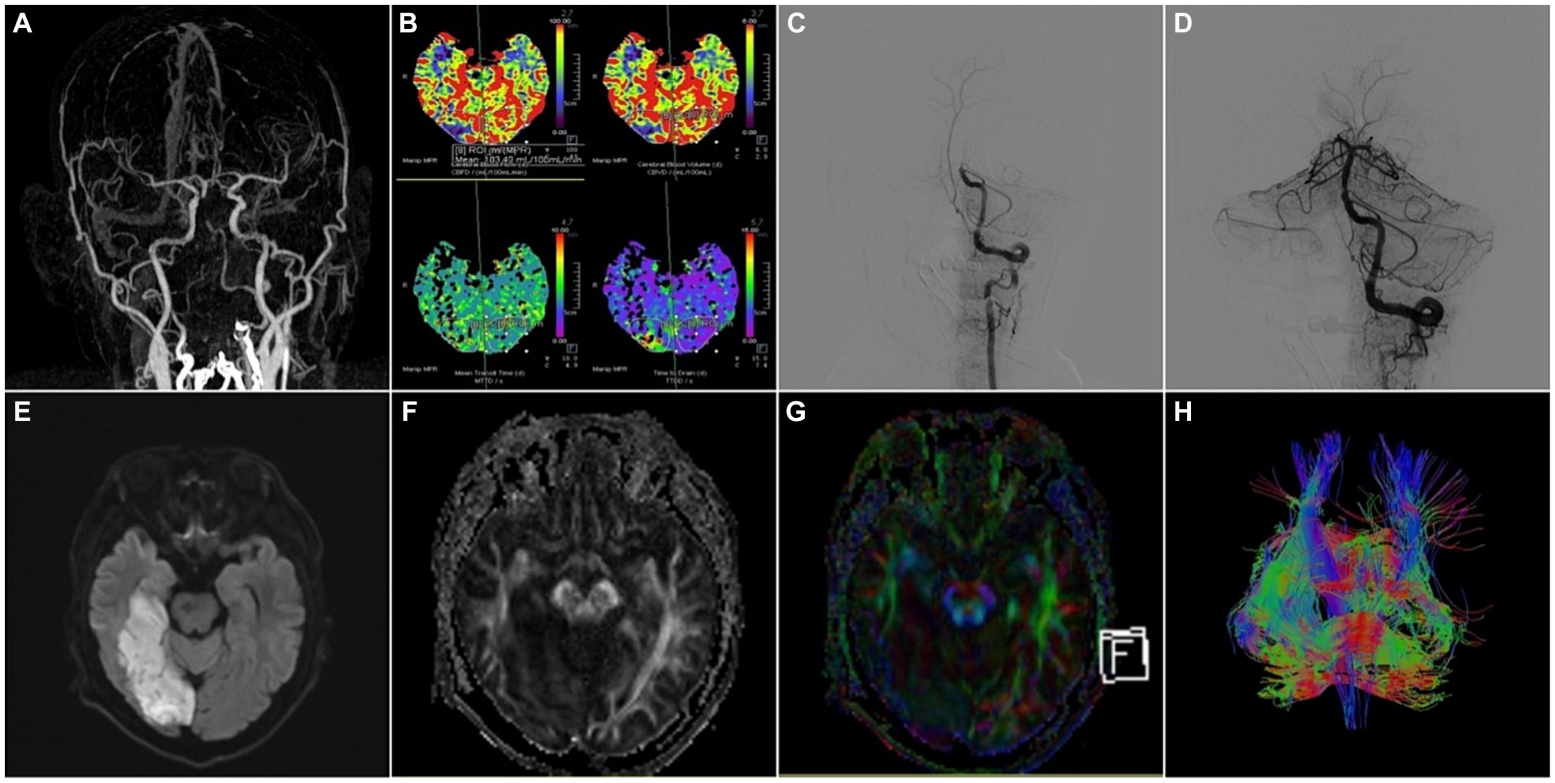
A 52-year-old male patient was admitted to the hospital with “weakness of left limb for 5 h” and was treated with intravenous thrombolysis by alteplase combined with arterial thrombectomy. (A) CTA-MIP: occlusion of the basilar artery; (B) PCT: the picture contained four important parameters of PCT like CBF, CBV, MTT and TTD, which showed the value of CBF, CBV became lower and the value of MTT, TTD became longer in the right occipital lobe compared to the left, that meant hypoperfusion in the basilar artery supply area; (C,D) DSA: occlusion of the basilar artery was demonstrated by left vertebral artery angiography, and the basilar artery was recanalized with moderate proximal stenosis after thrombectomy, the left posterior cerebral artery was patent, and the distal right posterior cerebral artery did not have significant visualization; (E) MRI-T2WI, (F) MRI-DTI-FA, (G) MRI-DTI-colorful FA, (H) MRI-DTI-fiber tractography: 4 days after thrombectomy, T2WI showed the right temporo-occipital lobe had a patchy infarct focus, and DTI demonstrated the right tractus pyramidalis were thinner than those on the left side.
